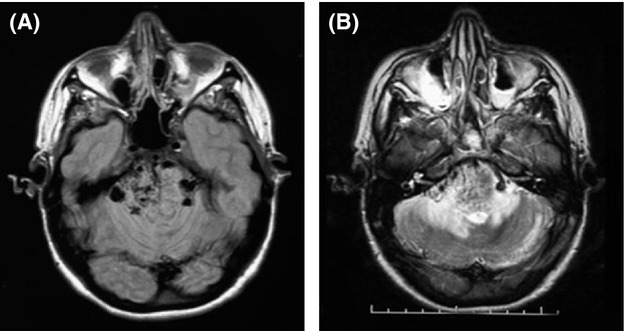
AVM located in right pons after gamma knife treatment. (A) Axial T1-weighted MRI brain. (B) Axial T2-weighted MRI brain. AVM, arteriovenous malformations; MRI, magnetic resonance imaging.
